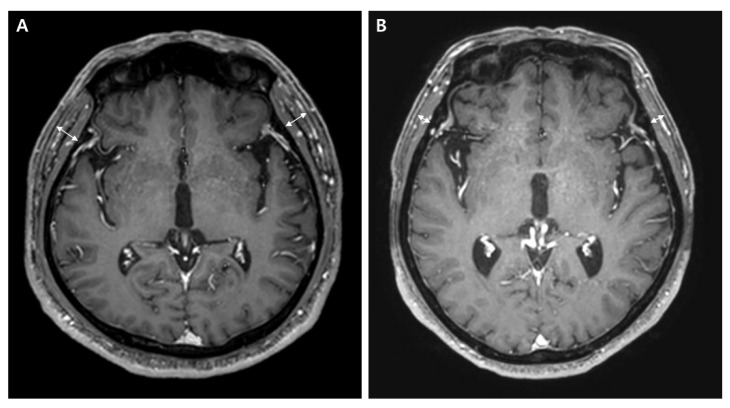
Representative cases of TMT measurements on brain MRI. (A) A 72-year-old male patient with an overall survival of 7 months (right TMT = 10.6 mm, left TMT = 11 mm, mean TMT = 10.8 mm) and (B) a 74-year-old male patient with an overall survival of 4 months (right TMT = 5.5 mm, left TMT = 6.2 mm, mean TMT = 5.9 mm).
